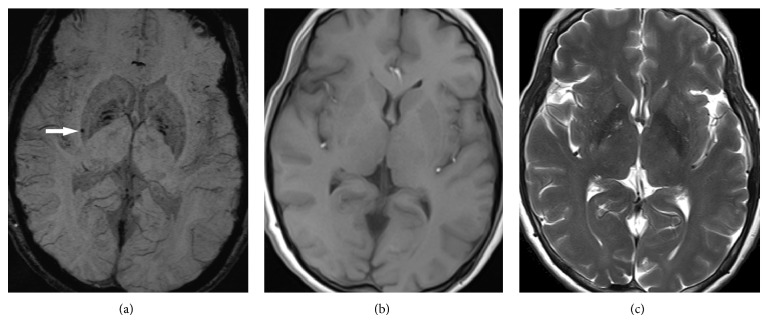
Magnetic resonance (MR) images of a 59-year-old man with end-stage renal disease. (a) SWI shows a hypointense lesion in the right putamen (arrow). (b) and (c) T1- and T2-weighted images do not show the corresponding lesion shown in (a).
